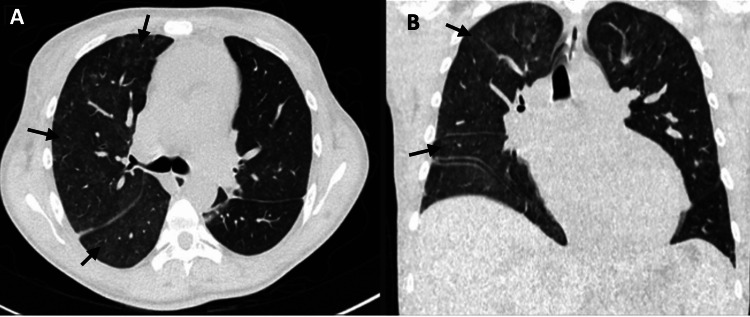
Chest CT lung window (A) Axial lung window. (B) Coronal reconstruction. Seventy-two hours after the initiation of immunosuppressive treatment, a few centroacinar ground-glass opacities of diffuse were observed.
